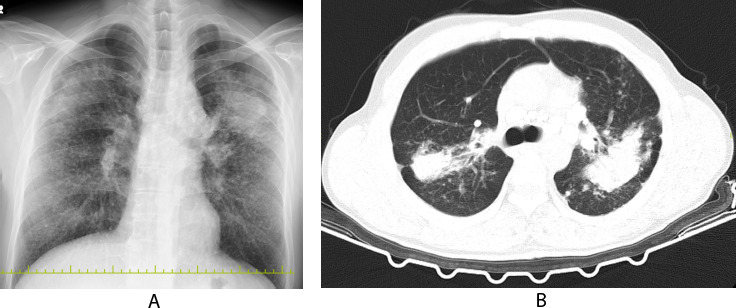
A: ILO pneumoconiosis PA lung X-ray classification q/q 3/3 + B opacity. B: Lung CT with small opacities and large opacity.
